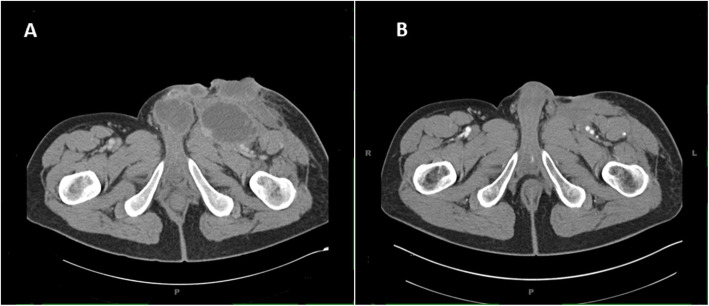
Computed tomography pelvis showing a peripherally enhancing low-attenuation lesions in the left lower pelvis extending into the proximal thigh with invasion of the adductor muscles and abutment of the penile shaft with possible invasion, there is also a subcutaneous lesion with ulceration and central necrosis. b Interval resolution of the previous low attenuating masses in the left inguinal region and the base of the penile shaft after initiation of pembrolizumab
